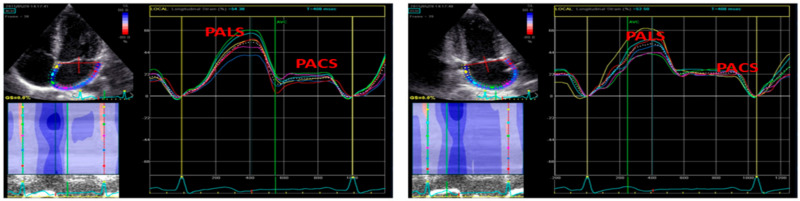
Speckle tracking echocardiography (STE) derived methodology to calculate left atrial (LA) strain in apical 4- and 2-chamber views. Left panel—upper left corner: two-dimensional echocardiography apical 4-chamber view, bottom left corner: color rendering of LA strain variation during cardiac cycle, right side: LA strain curves with peak atrial longitudinal strain (PALS) and peak atrial contraction strain (PACS). Right panel—upper left corner: two-dimensional echocardiography apical 2-chamber view, bottom left corner: color rendering of LA strain variation during cardiac cycle, right side: LA strain curves with peak atrial longitudinal strain (PALS) and peak atrial contraction strain (PACS).
